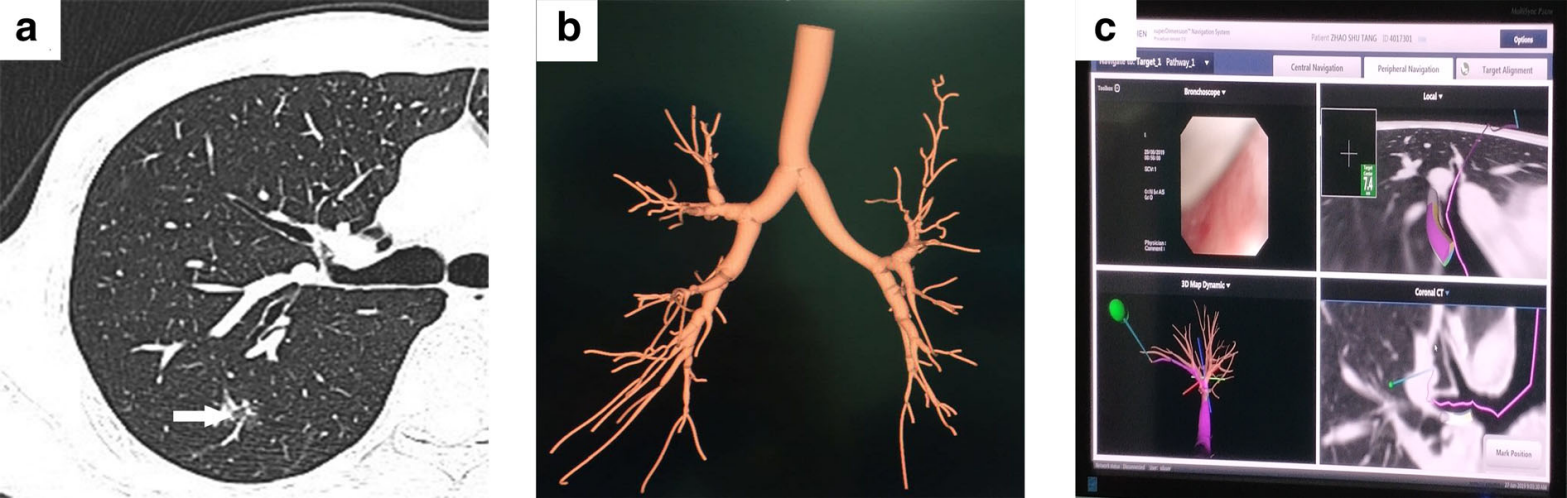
(a) Chest computed tomography (CT) showed a ground‐glass opacity (GGO) (white arrow) located in the right S^2^a subsegment. (b) A 3‐dimensional reconstructed depiction of the bronchial tree using electromagnetic navigational bronchoscopy (ENB) software. (c) Shows the real‐time navigation screen when the ENB‐guided dye marking method was performed
